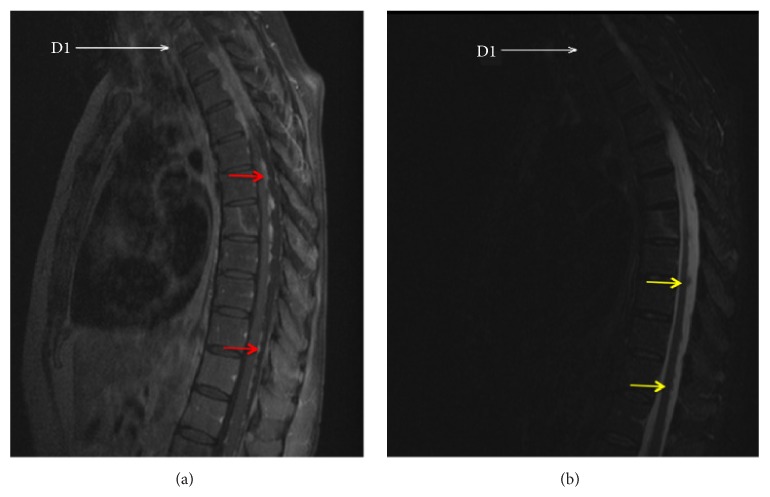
(a) Sagittal gadolinium-enhanced T1-weighted Turbo Spin Echo Fat Sat MR image. Multiple round enhancing foci (red arrows) sticking to the posterior medulla, corresponding to diffuse leptomeningeal spread of metastases. (b) Sagittal T2 Short Time Inversion Recovery (STIR) MR image. The leptomeningeal metastases are also well depicted as hipointense nodules on the STIR sequence (yellow arrows) surrounded by the T2-hyperintense CSF.
